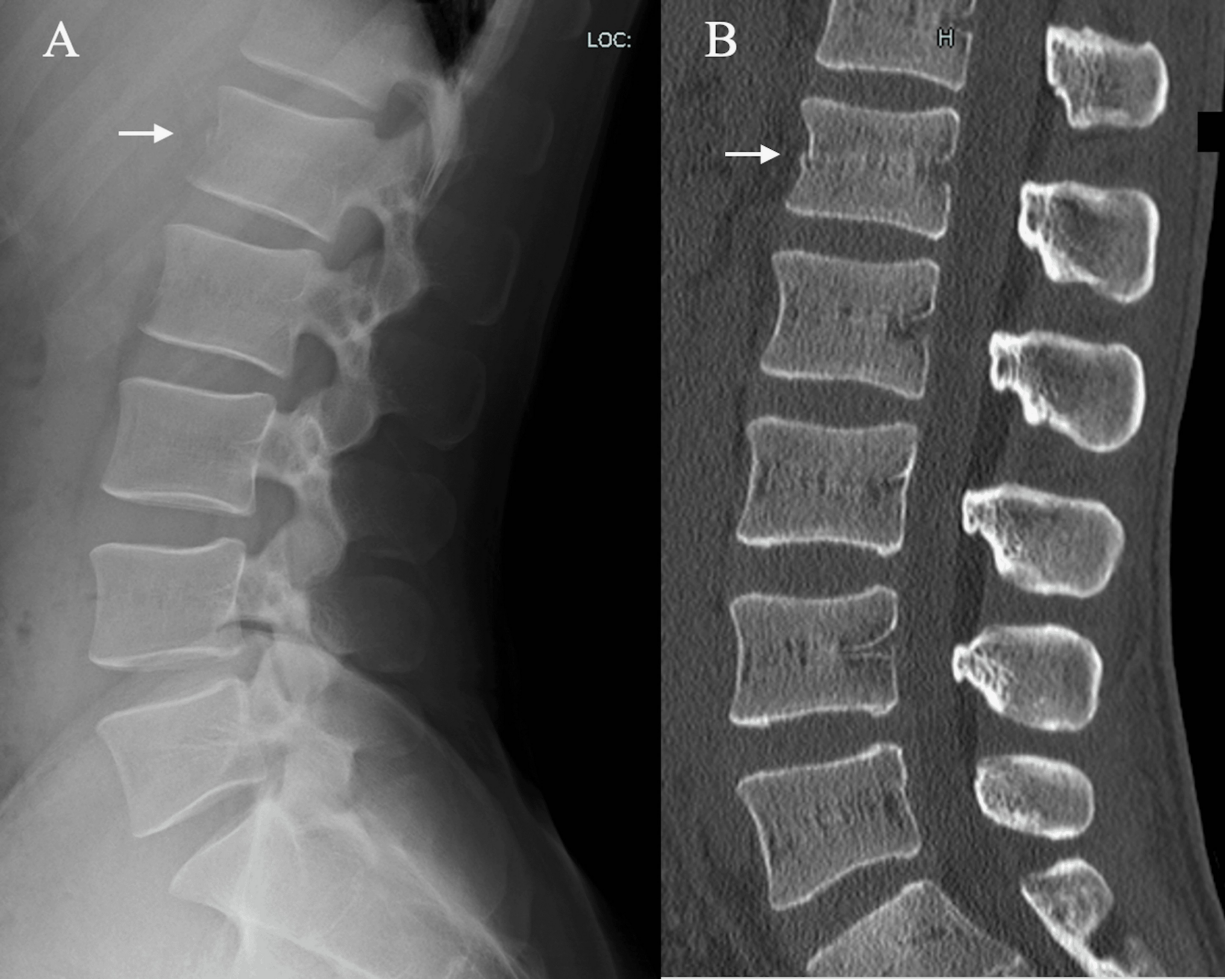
Findings at the day after injury (A) Lateral radiograph showing a fracture of the L1 vertebra. (B) CT sagittal view of the spine showing a fracture of the L1 vertebra (white arrows). CT: computed tomography
